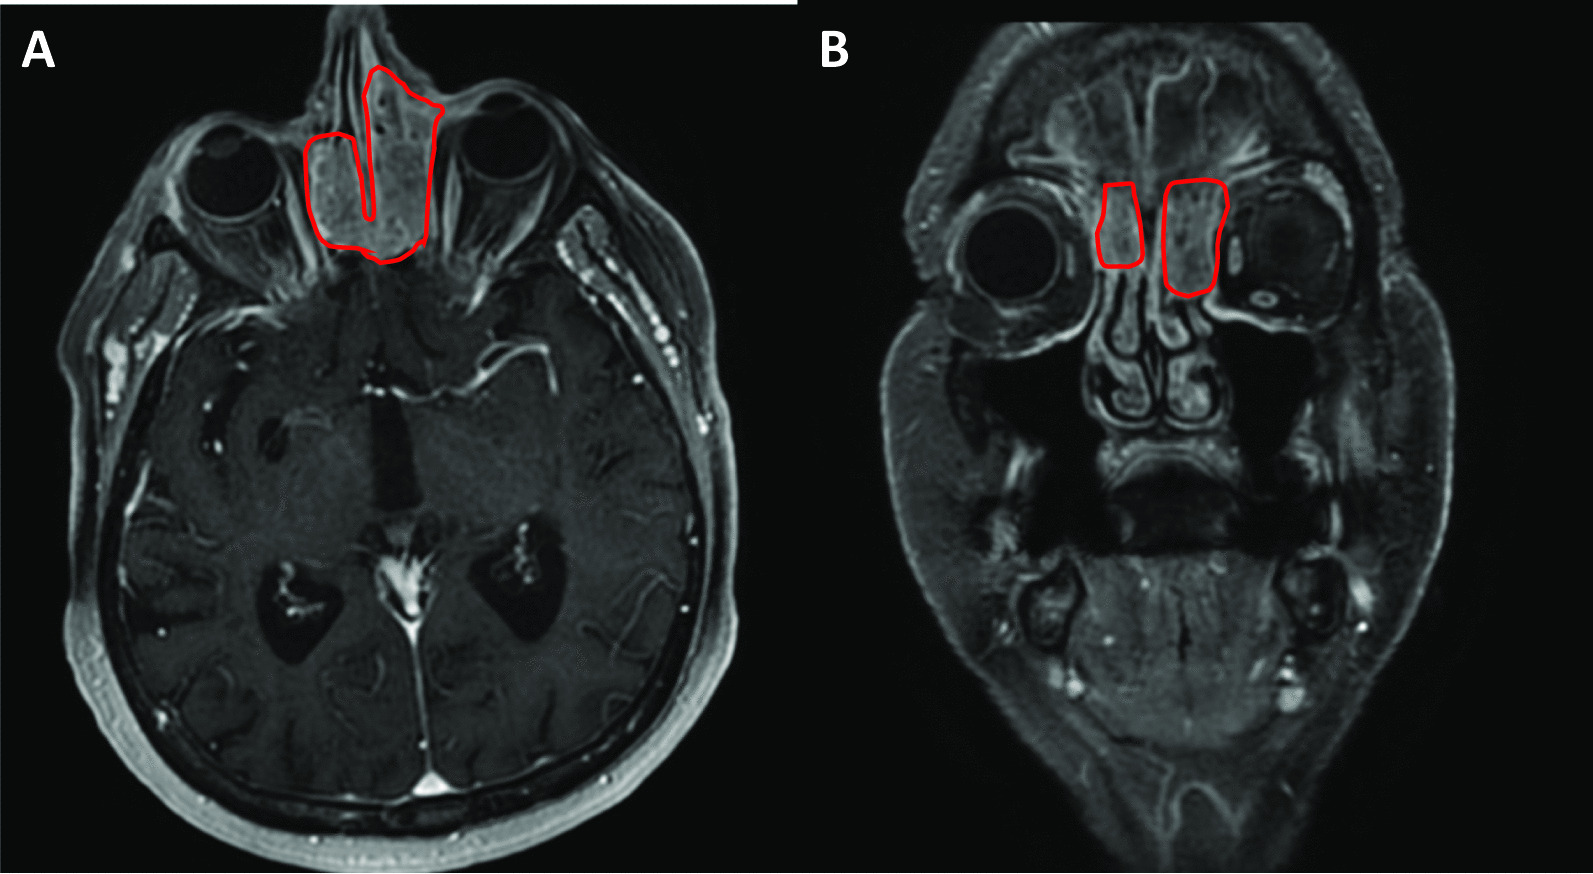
A, B MRI scans of the facial skeleton indicating the spread of the BCC into the nasal and frontal sinuses, as well as into the ethmoidal air cells in the left regions of the inner canthus and nasal root (April 2018)
